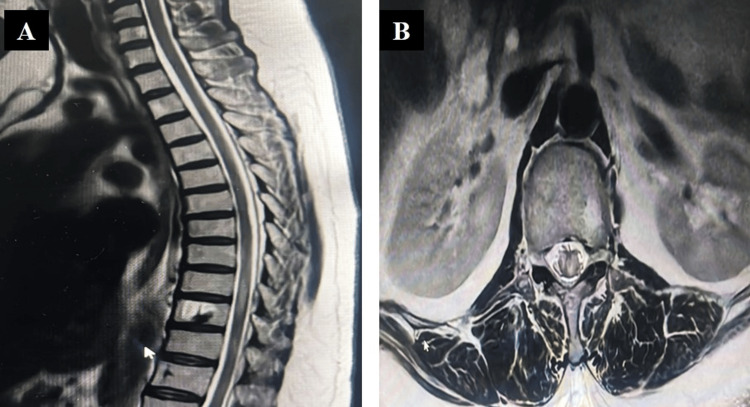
(A) MRI of the dorsolumbar spine revealed myelitis at D9-10 levels in the sagittal section. (B) Myelitis evident in the axial section.
